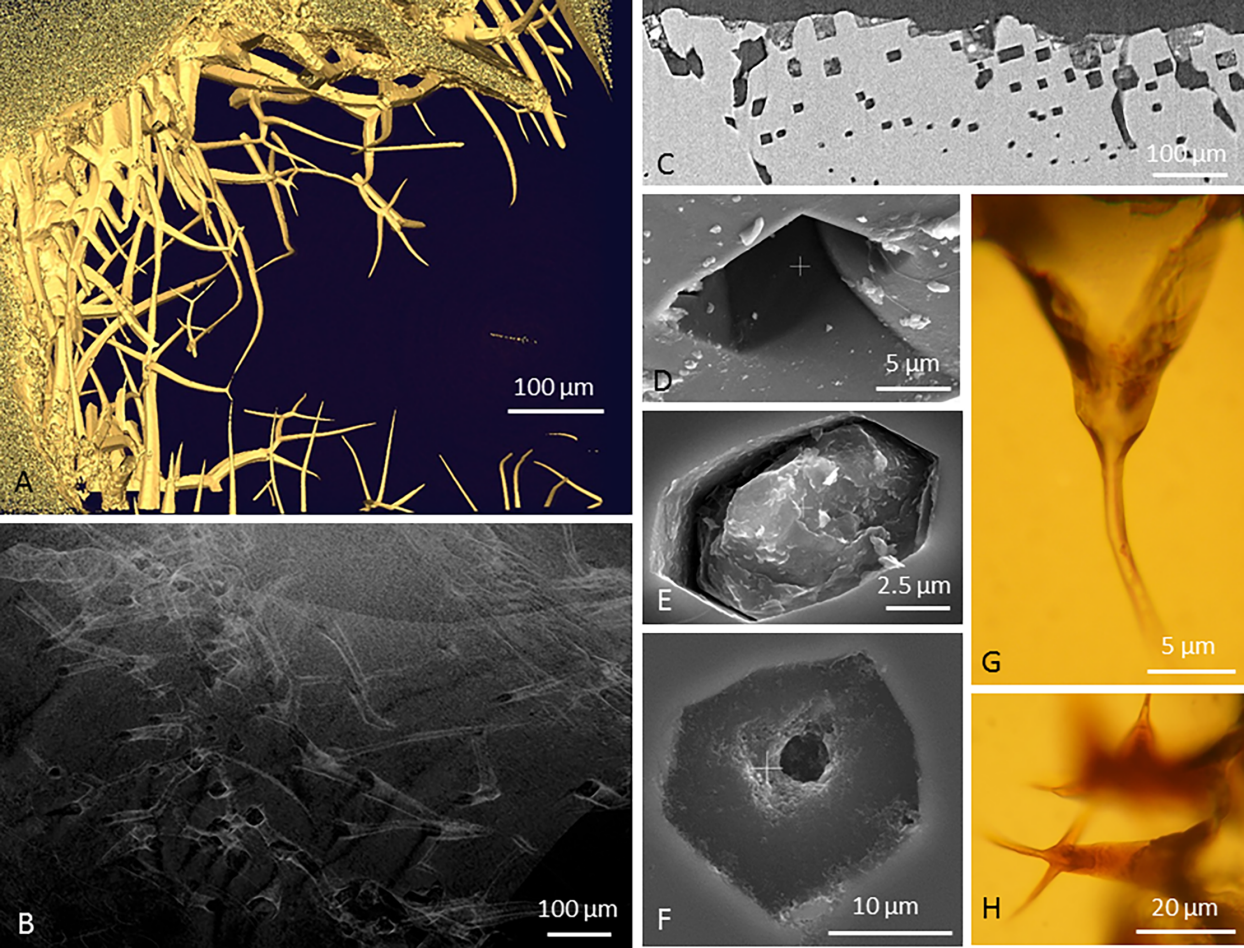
Images A-B: CT almandines; C-F: KW pyropes; G-H: CT pyropes. A) Tomographic reconstruction (isosurface rendering) of a garnet with tubular structures originating at the surface. The bases of the tunnels are broad and have distinct hexagonal or rectangular cross sections but tapering off and become more rounded toward the tips. B) Tomographic reconstruction (volumetric rendering) showing the hexagonal cross section of multiple tubular structures. C) An orthoslice of a tomographic reconstruction showing the cross-sectional hexagons or rectangles of the tunnels. D) SEM image of a four-angled polygonal entrance hole. E) SEM image of a six-angled polygonal entrance hole that is filled. F) SEM image of a tubular structure that tapers off further into the mineral. Note how the tunnel have a polygonal shape at the mineral surface but further in gets more circular as it tapers off. G) Microphotograph of a tubular structure that tapers off and also starts with a polygonal shape at the mineral surface but gets more circular as it penetrates further into the mineral and tapers off. H) Microphotograph of tubular structures that tapers off. The branching of the tunnels results in offspring tunnels with less diameter than the originating tunnel.
